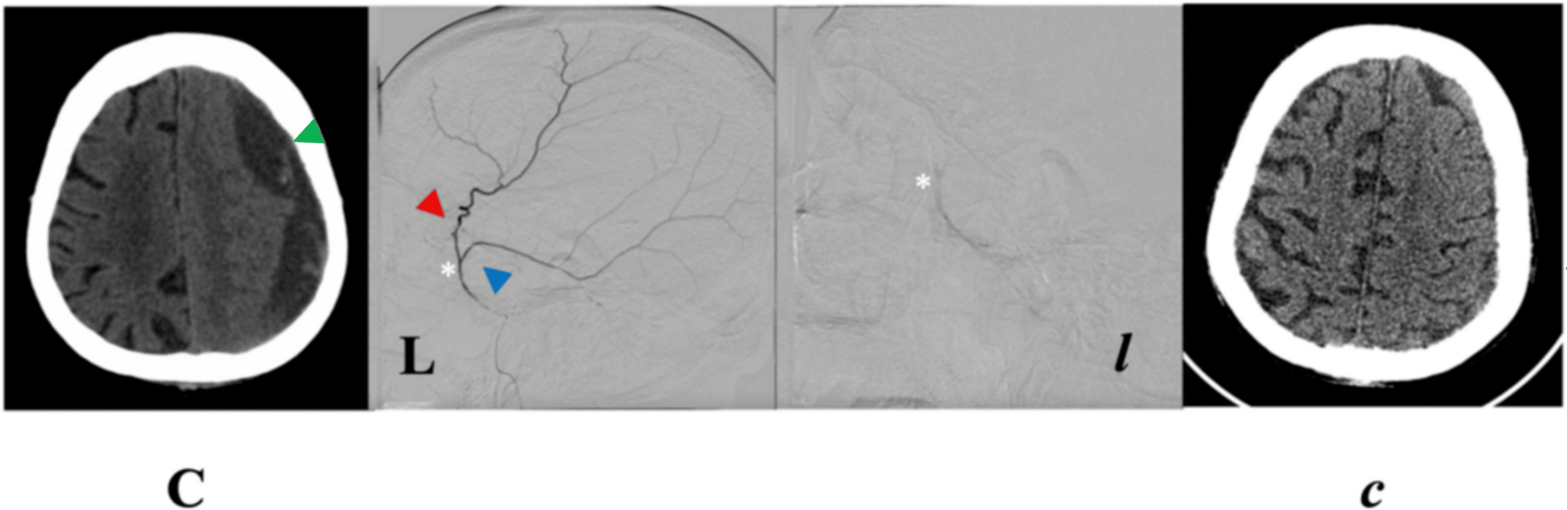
Case C. Pre-EMMA (C) and respective post-EMMA (C) cranial NCCT axial views at levels of maximum CSDH thickness. Pre-EMMA angiography in left lateral projection (L) shows the course of anterior and posterior MMA branches, and post-EMMA obliteration of these vessels (l). Angiographic projections correspond to contiguous NCCTs. EMMA, embolization of the middle meningeal artery; MMA, middle meningeal artery; NCCT, non-contrast computed tomography; R, right. Green arrowhead, chronic subdural hematoma; red arrowhead, anterior branch; blue arrowhead, posterior branch; white asterisk, MMA bifurcation point.
